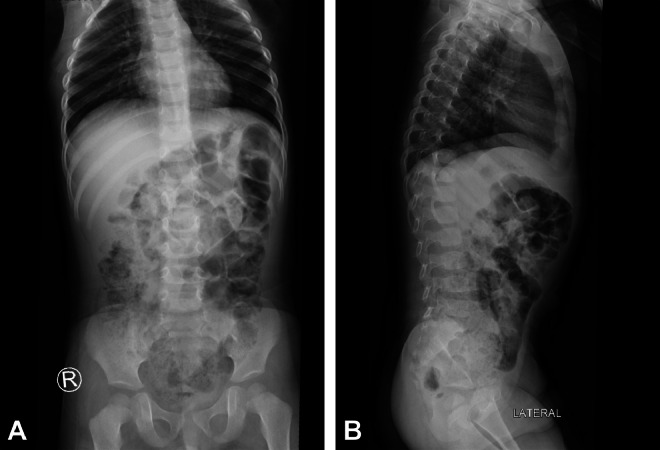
Supine anteroposterior (A) and lateral (B) X-ray images exhibit unremarkable findings.
